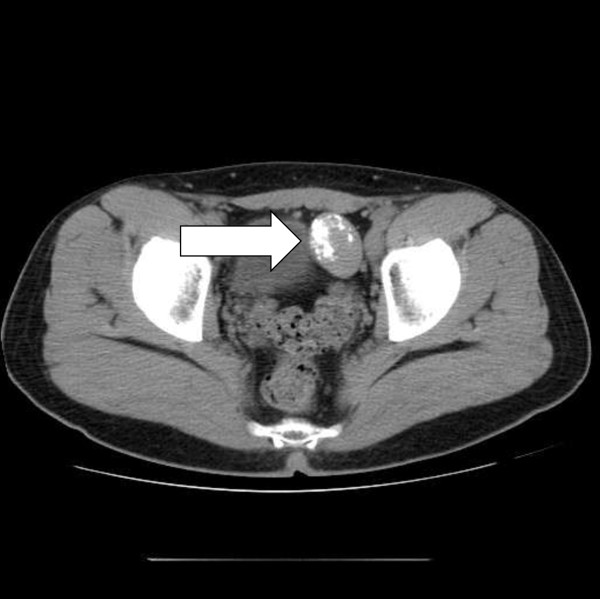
CT image of CFT in the pelvis.
